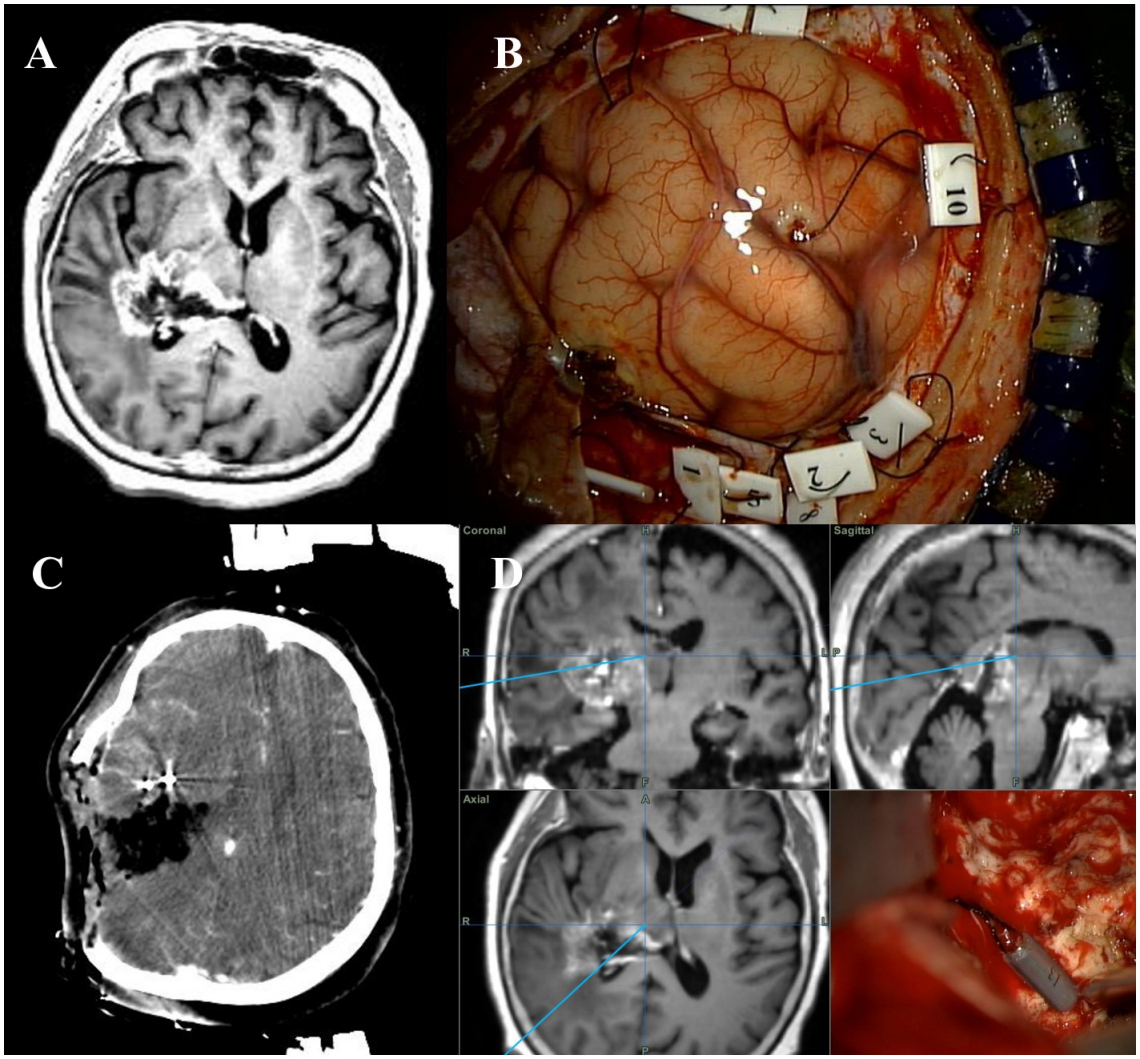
Adjuvant surgical technique. Preoperative magnetic resonance image of a glioblastoma of the right posterior thalamus with lateral extension (A). The tailed bullets are inserted into the target areas (B). During the operation, intraoperative computed tomography image and the tailed bullet technique are used for enabling adjustment for brain shifting and the confirmation of target lesion (C, D).
